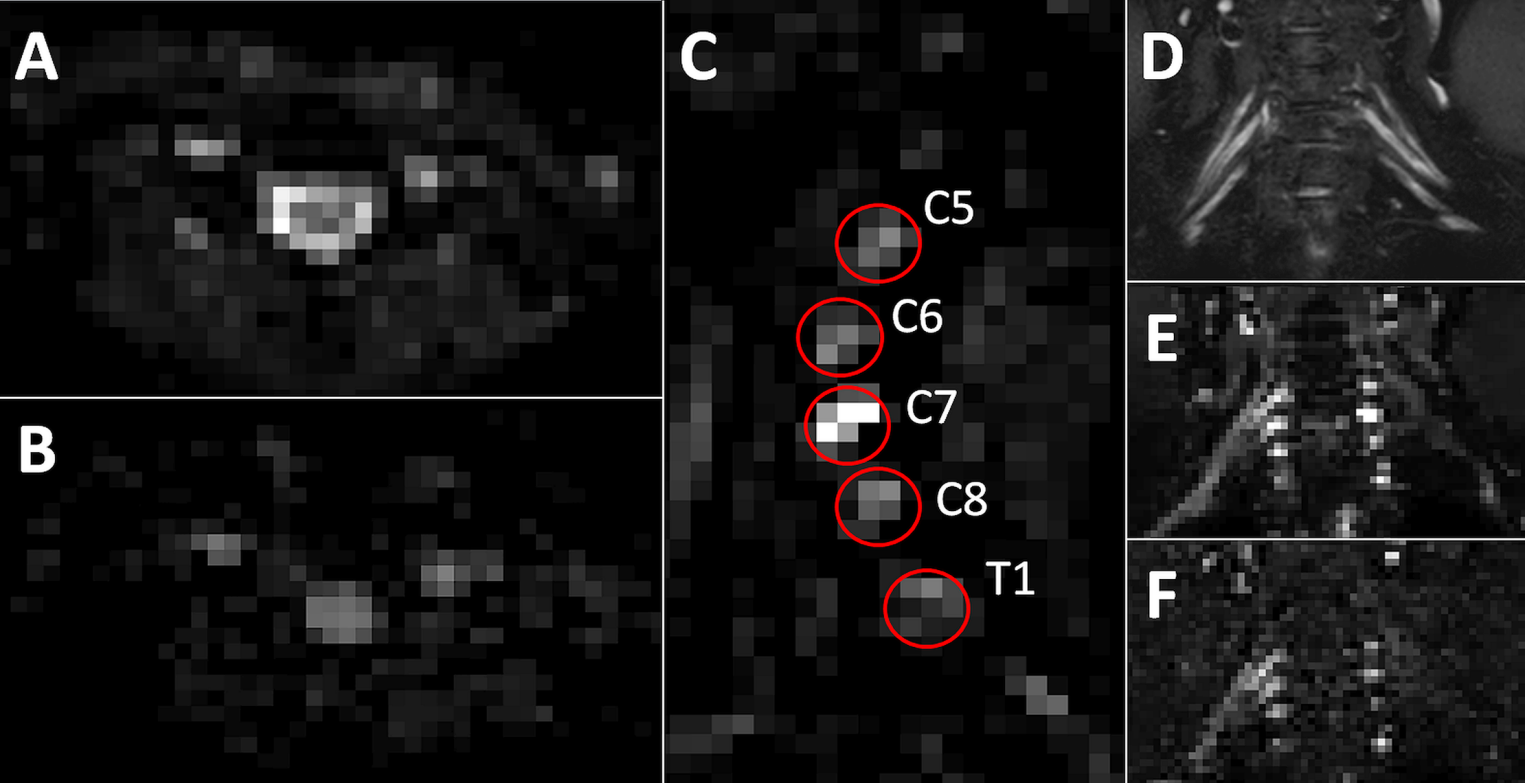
Diffusion and non-diffusion weighted images and segmentation. (A) DT-MRI with b-value = 0 in axial orientation. (B) DT-MRI with b-value = 800 s/mm^2^ in axial orientation. (C) Sagittal view of DT-MRI with b-value = 0 in which the red circles indicate the region of interest (ROI) placements per root. (D) Coronal MR neurography image with visualization of the roots and trunks. (E) DT-MRI with b-value = 0 reconstructed in coronal orientation. (F) DT-MRI with b-value = 800 s/mm^2^ reconstructed in coronal orientation.
